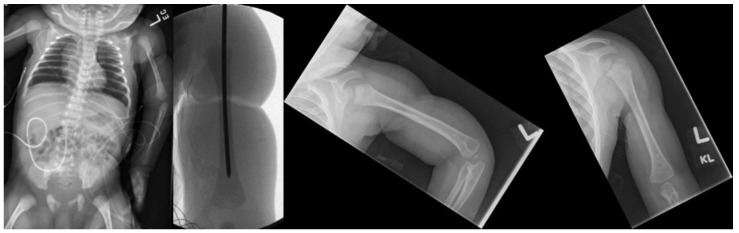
Radiographs of Patient 2: preoperative AP view, intraoperative AP view, and 1-year postoperative AP views of flexed and extended left upper extremity (left to right).
